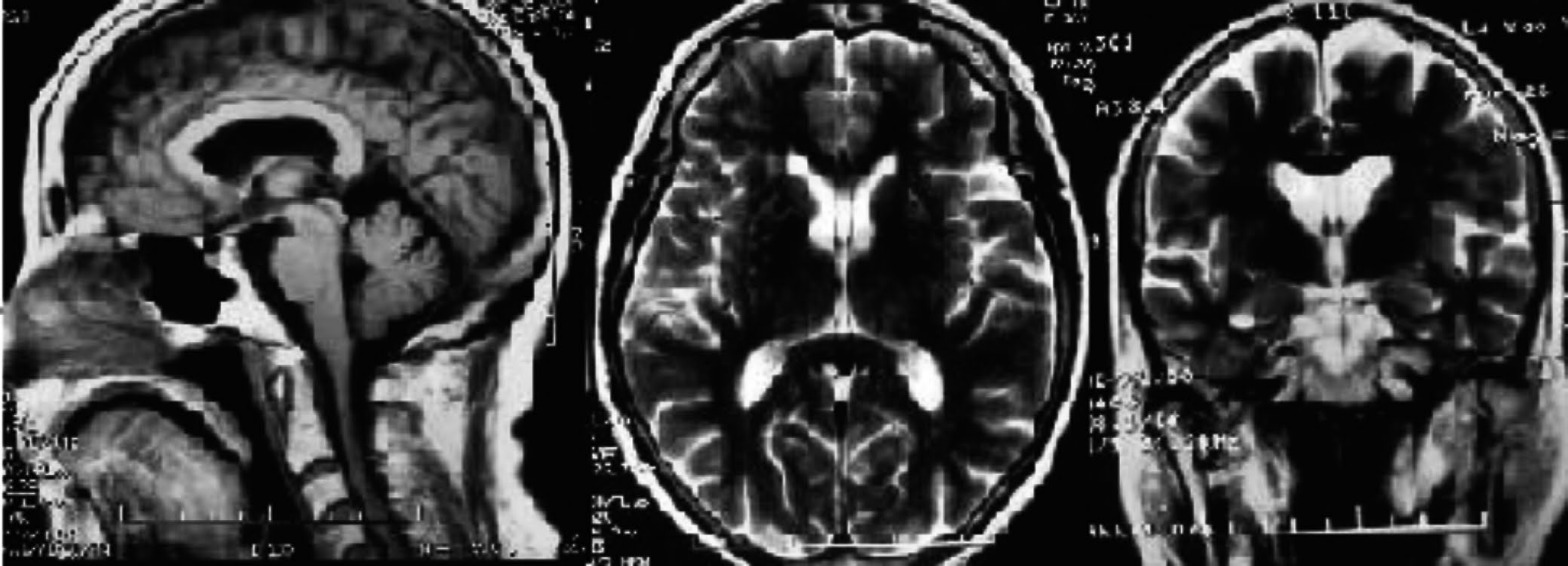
MRI images showing bilateral putamen atrophy and putaminal hyperintensive rim in one MSA‐P patient
